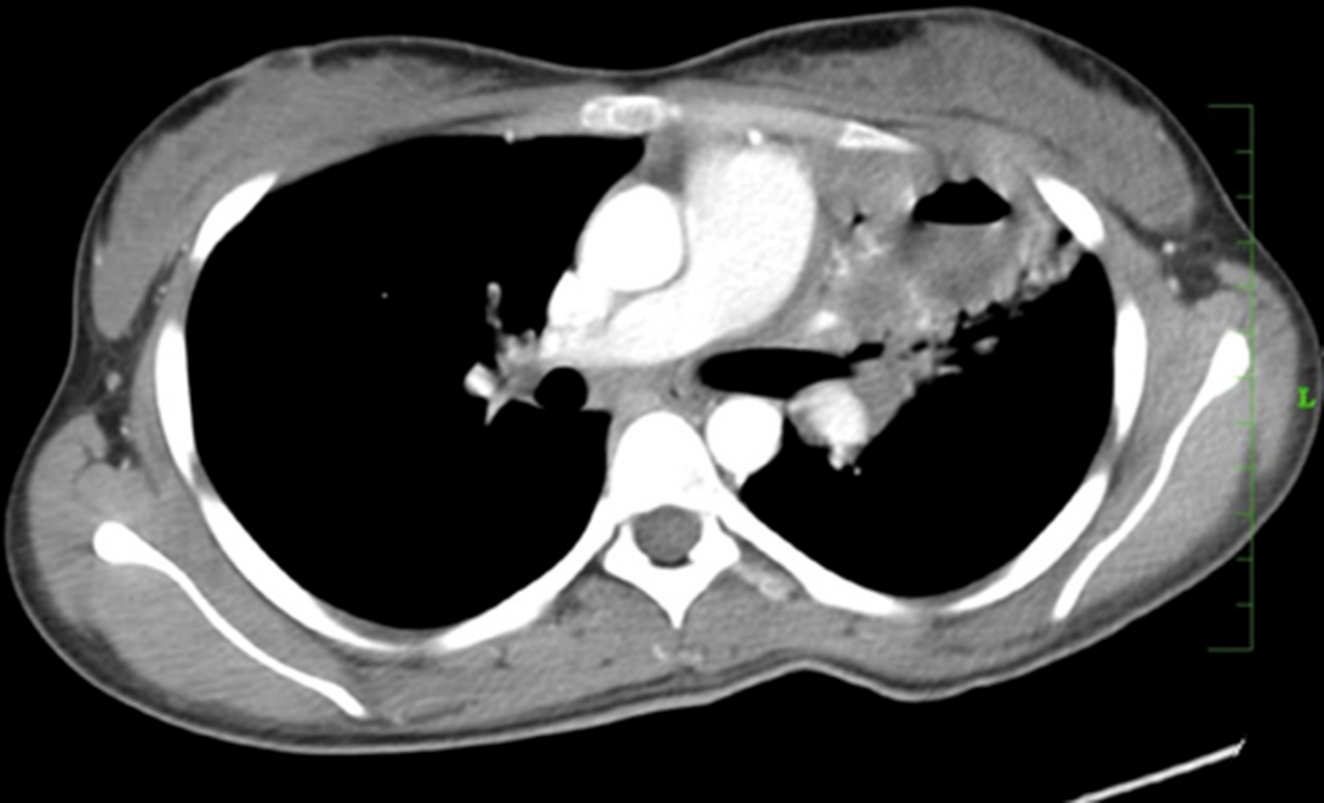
Case 2(b): Axial post-contrast CT image of the chest in mediastinal window shows cavitating consolidation in the left upper lobe with extension into the hilum.
